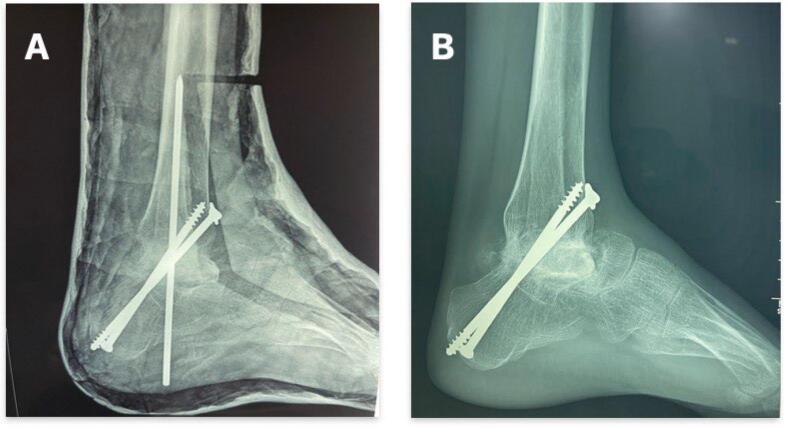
Post-operative X-ray of the left ankle; (A) at 3 months; (B) at 6 months.
